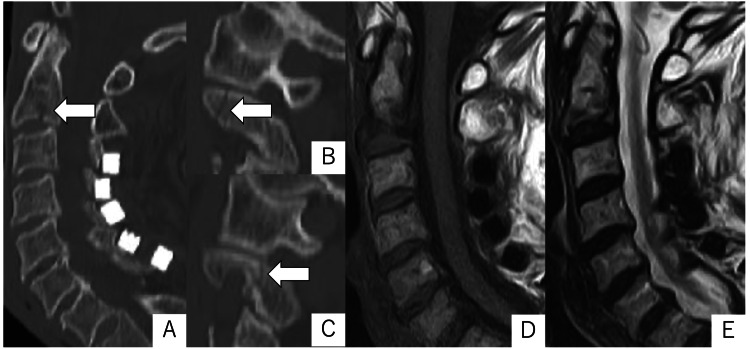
Sagittal CT images (A: midline, B: right, C: left) and magnetic resonance (MR) images (D: T1W, E: T2W) obtained in the early days after the injury. CT images showed fracture lines in the C2 pedicle and vertebral body (arrows). MR images showed signal changes in the vertebral body, but no evidence of disc or ligament injury. T1W: T1-weighted; T2W: T2-weighted.
